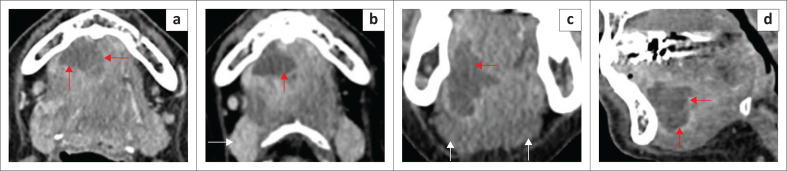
A 69-year-old male patient presented with a painful swelling in the right sublingual space, difficulty in swallowing and pain in the submandibular region. Contrast-enhanced CT (a to d) demonstrates an irregular rim-enhancing abscess (red arrows). The axial image (b) shows mild borderline enlargement with increased enhancement of the right submandibular gland (white arrow). The abscess is located above the mylohyoid muscle (c, white arrows).
